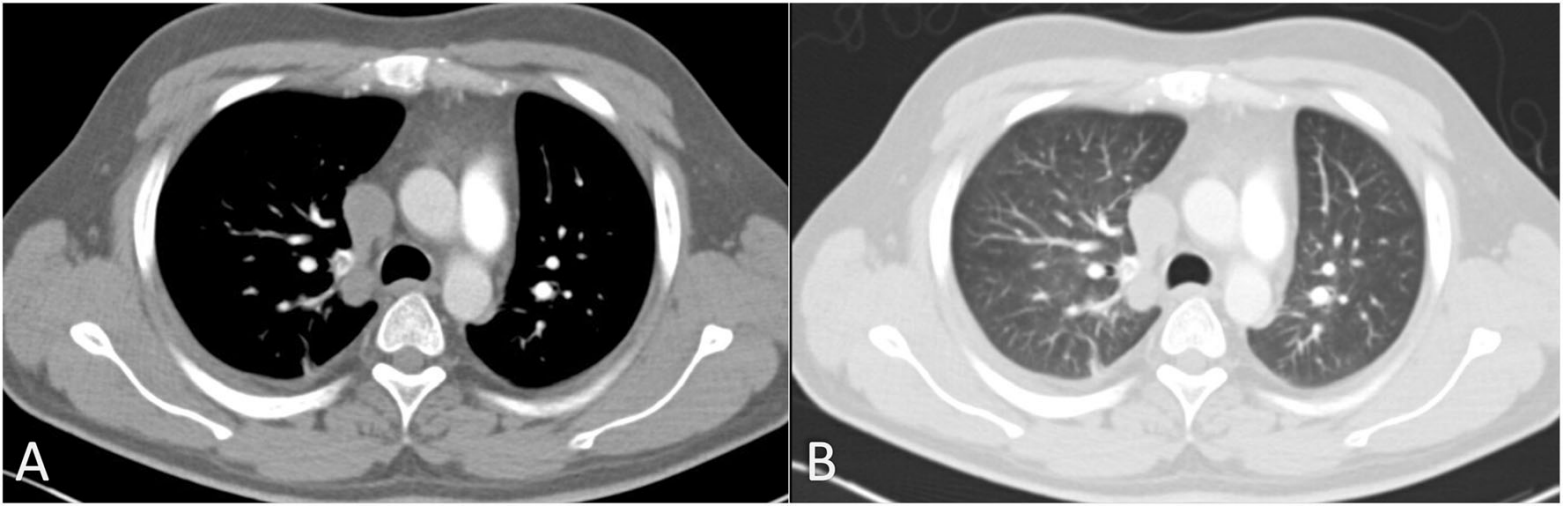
Axial computed tomography pulmonary angiography images in the pulmonary thromboembolism-specific (a) and lung (b) windows, from a 31-year-old man with sickle cell disease presenting with dyspnea, demonstrating a filling defect within the truncus anterior (a) and peripheral parenchymal atelectasis (b)
